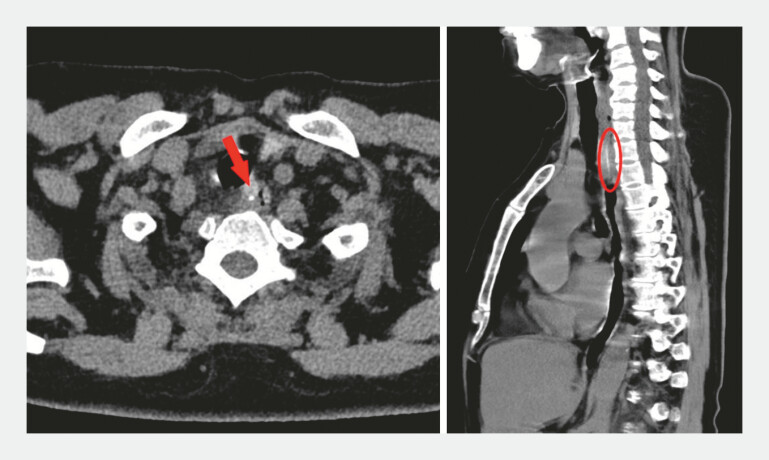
A preoperative computed tomography (CT) scan of the chest.
